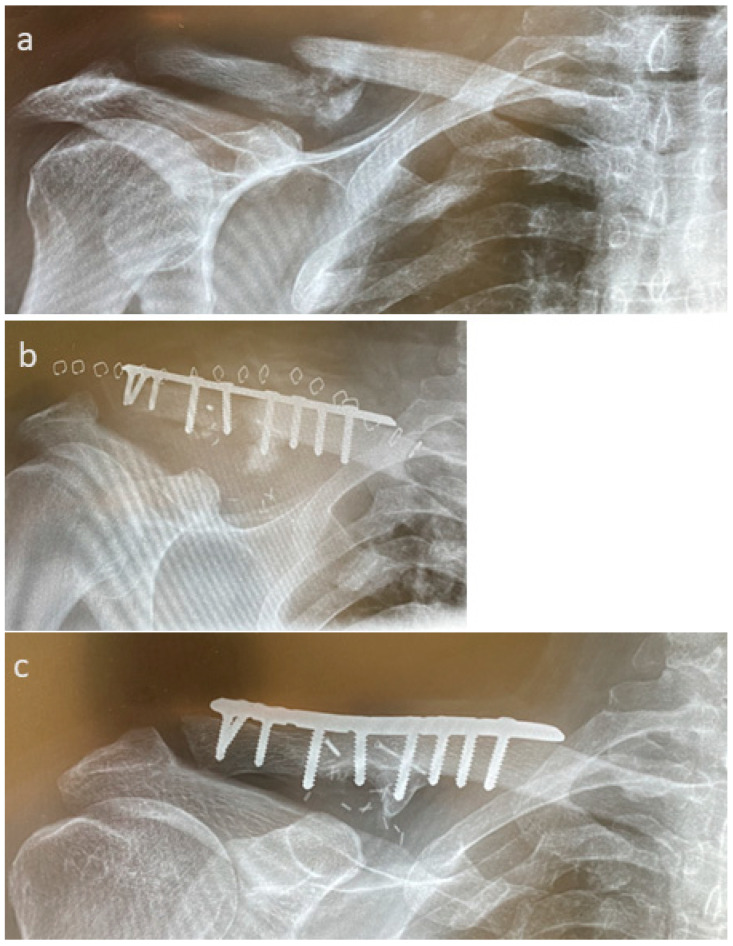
A case of non-union after a patient faced osteomyelitis after a fall at home. (a): Presurgical X-ray. (b): The fracture was anatomically reduced after pseudoarthrosis removal, the insertion of a microvascular pedicled MFC span, and bridging osteosynthesis with a 10-hole lateral clavicle plate (Arthrex, Naples, FL, USA). (c): Complete union one year after revision.
